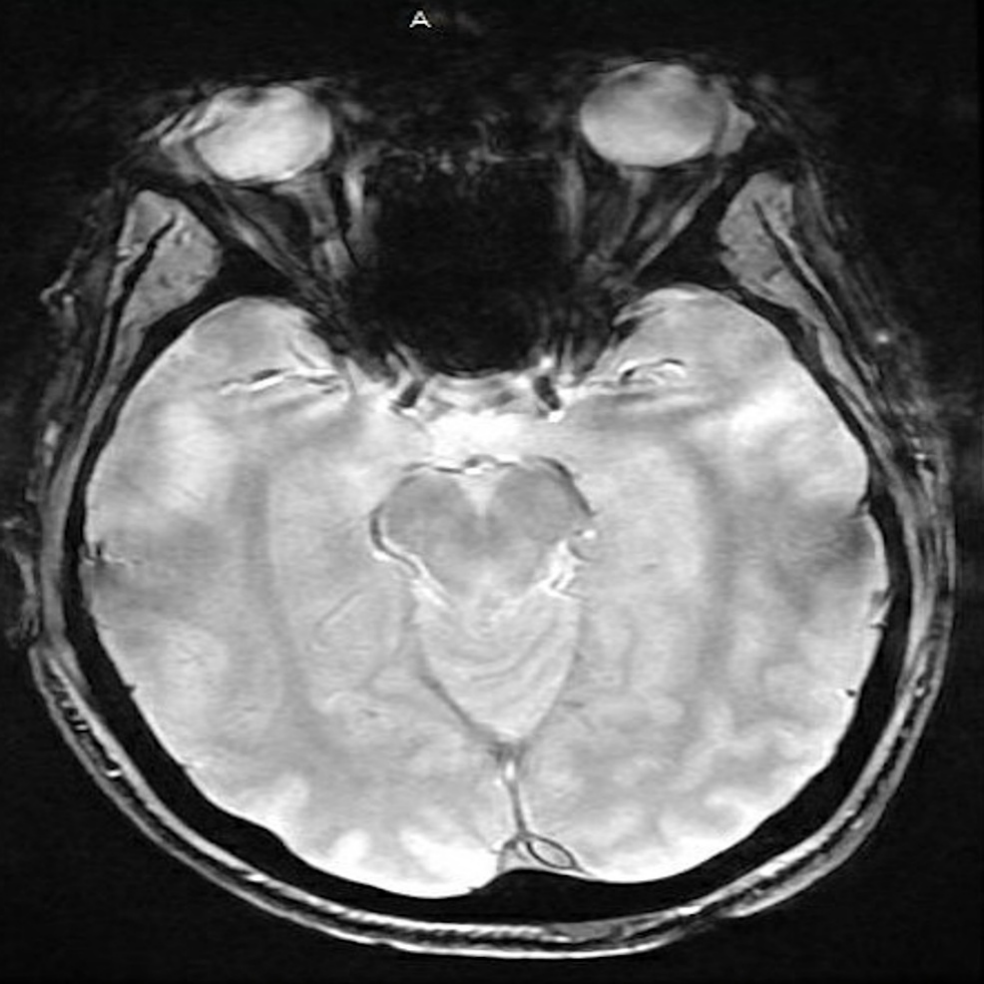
MRI of the brain. Unremarkable MRI scan of the brain showing no intracranial pathology.
